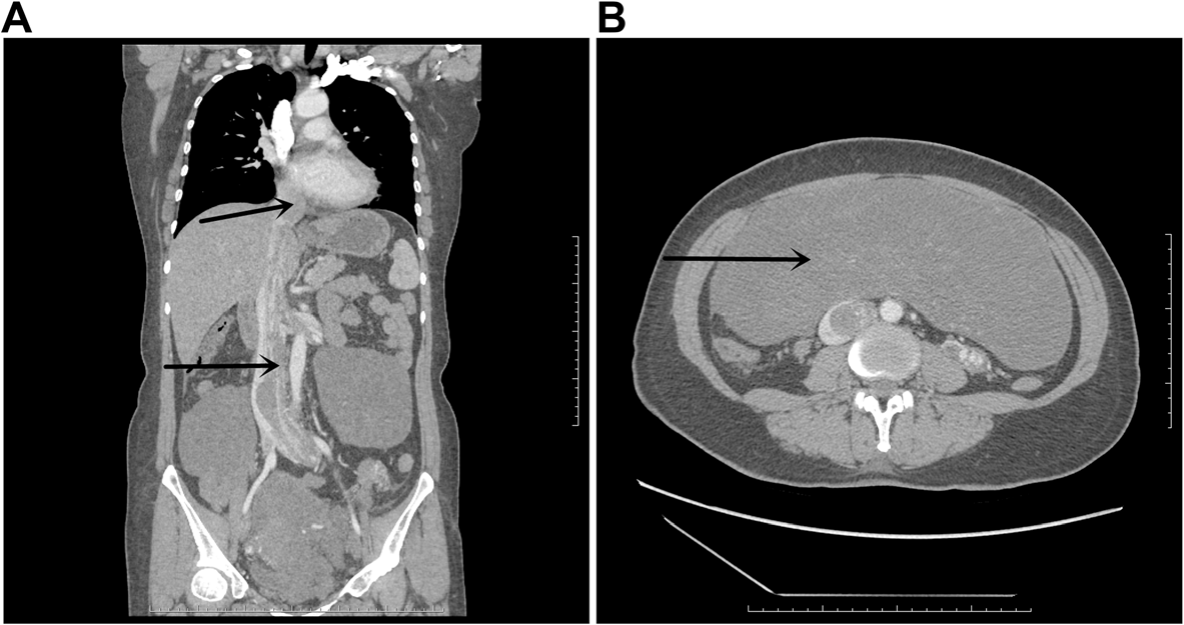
Enhanced computerized tomography (CT) shows the tumor encroached on the IVC, right atrium and right ventricle (a
